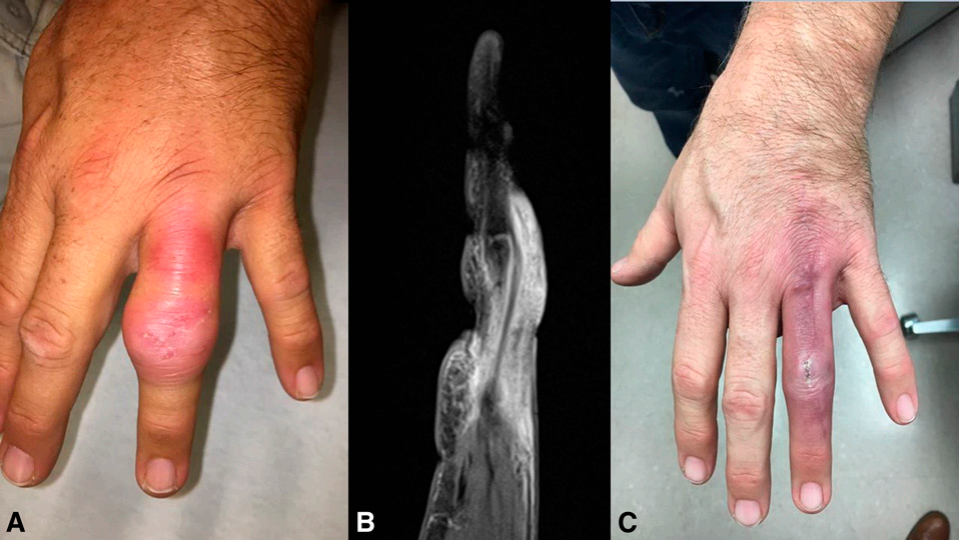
(A) Tenosynovitis of the ring finger. (B) Magnetic resonance imaging of the finger. (C) Appearance of the finger after 3 months of antibiotic treatment. This figure appears in color at www.ajtmh.org.
